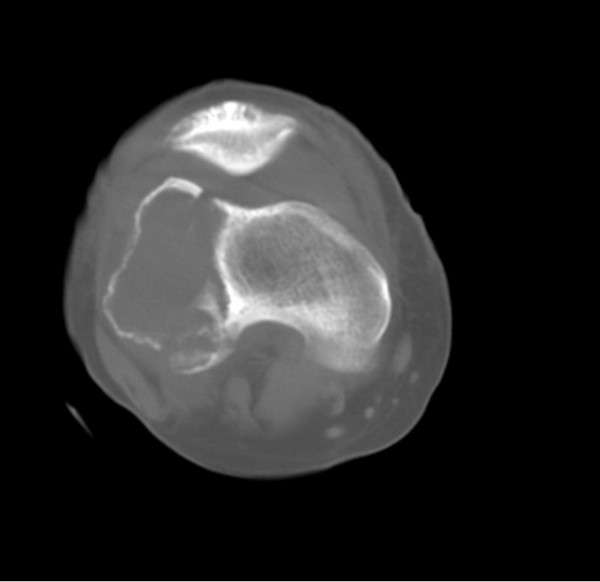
CT Scan of Knee Joint showing Tumor extent.
